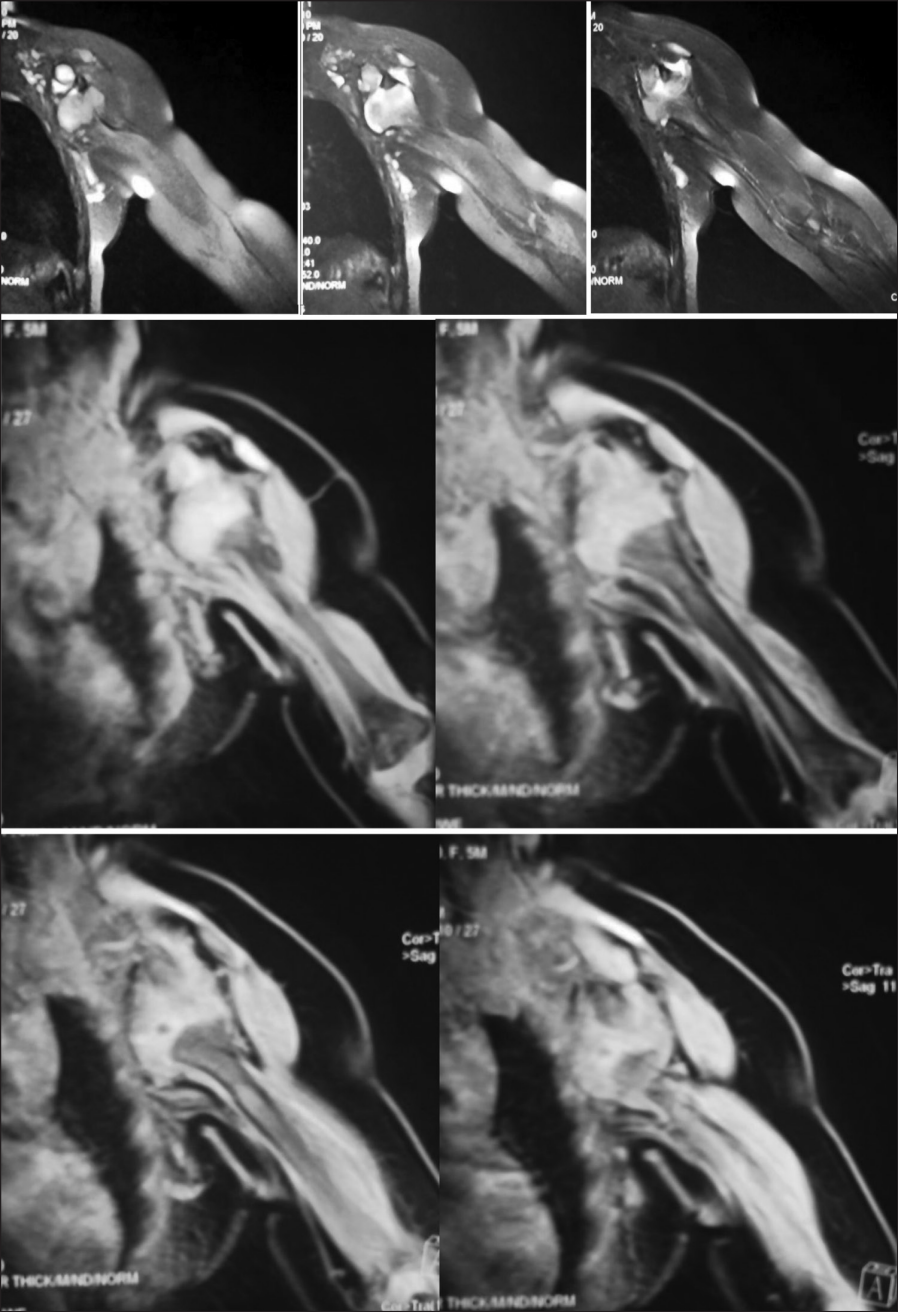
MRI images of the left shoulder showing an anteroinferior dislocation
